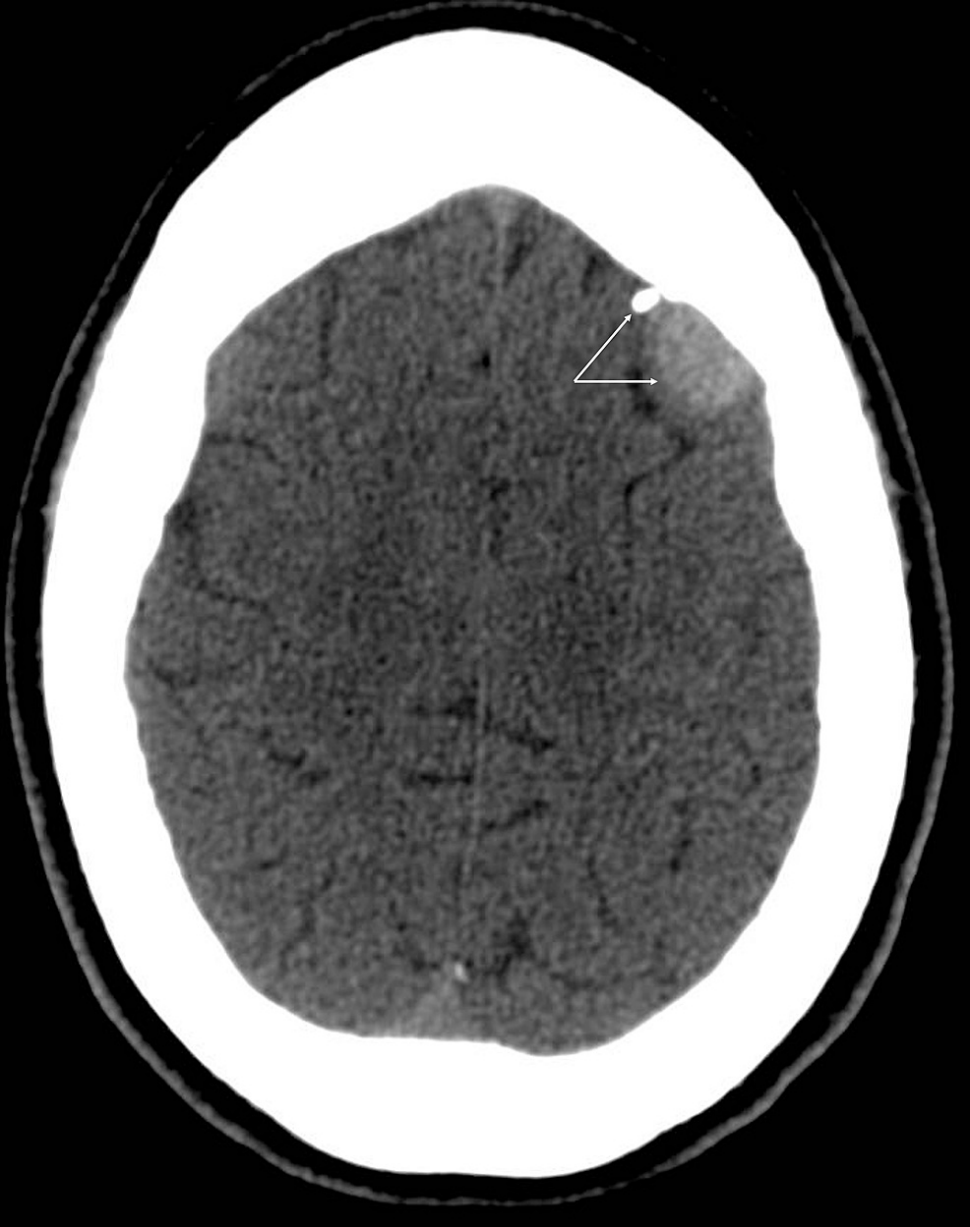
Initial CTH demonstrating the lesion. Non-contrasted CTH with axial view demonstrating a round hyper-dense extra-axial lesion in the left frontal cortex; there is an associated calcium deposit suggestive of calcified meningioma (arrows). Sagittal and coronal sections are not shown due to poor image fidelity impeding MPR. CTH: CT scan of the head; MPR: Multiplanar reformation.
